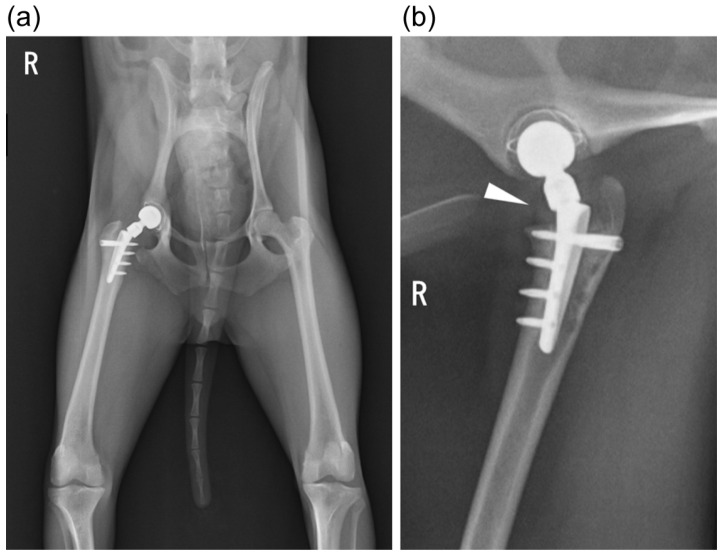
Postoperative radiographs after first total hip replacement surgery (right hip). (a) Ventrodorsal view; (b) yoga view: the stem was placed slightly proximally, as indicated by the arrowhead.
